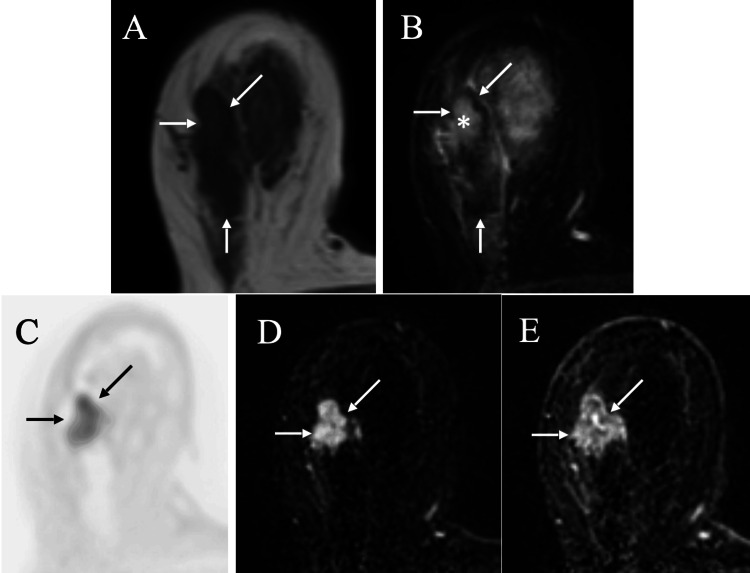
Magnetic resonance imaging (MRI) findings MRI of the target lesion showed low signals (arrows) on T1-weighted images (A), focal high signals (asterisk) in the large low signals (arrows) on fat-suppressed T2-weighted images, focal high signals (arrows) on diffusion-weighted images (C), and focal both early (D, arrows) and retained (E, arrows) enhancement on dynamic studies.
